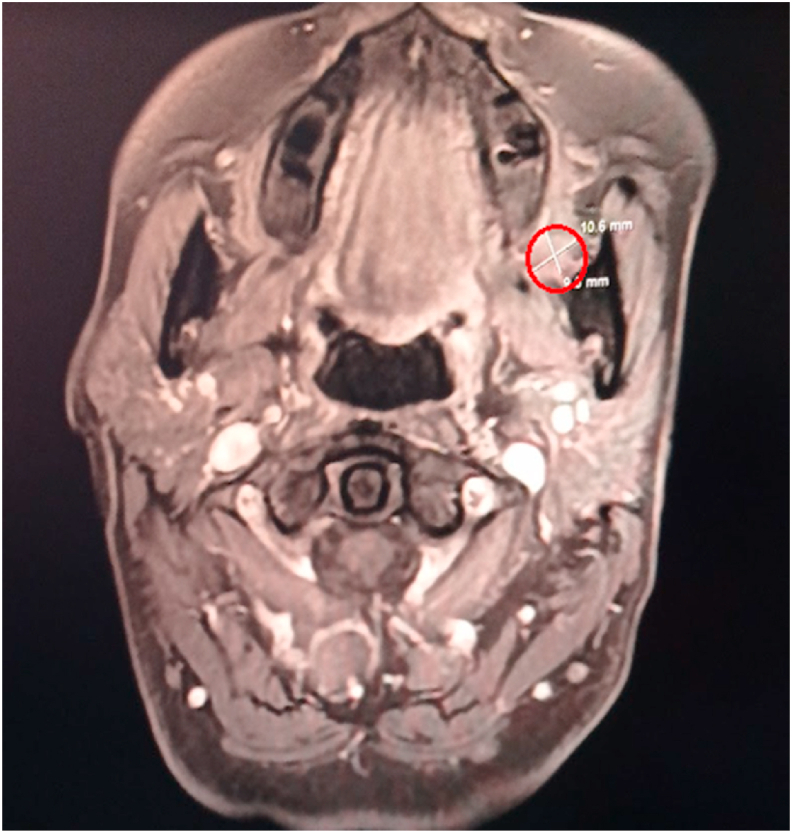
MRI axial section revealing a soft tissue tumor in the left retromolar region.
